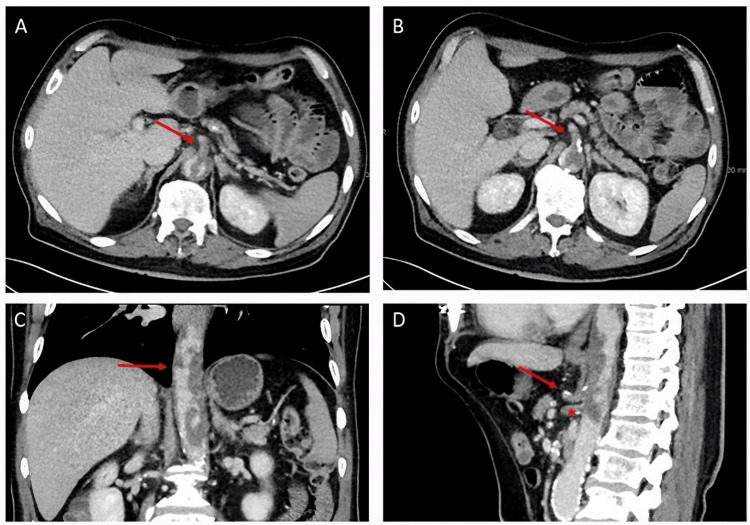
Contrast-enhanced CT imaging demonstrating thrombosis of the superior mesenteric artery (SMA), the celiac trunk, and the descending and abdominal aorta. A: Axial contrast-enhanced CT scan showing thrombosis of the SMA (arrow); B: Axial contrast-enhanced CT scan showing thrombosis of the celiac trunk (arrow); C: Coronal contrast-enhanced CT scan demonstrating thrombosis of the descending and abdominal aorta (arrow); D: Sagittal contrast-enhanced CT scan showing thrombosis of the celiac trunk (arrow) and the SMA (asterisk). CT: computed tomography; SMA: superior mesenteric artery.
